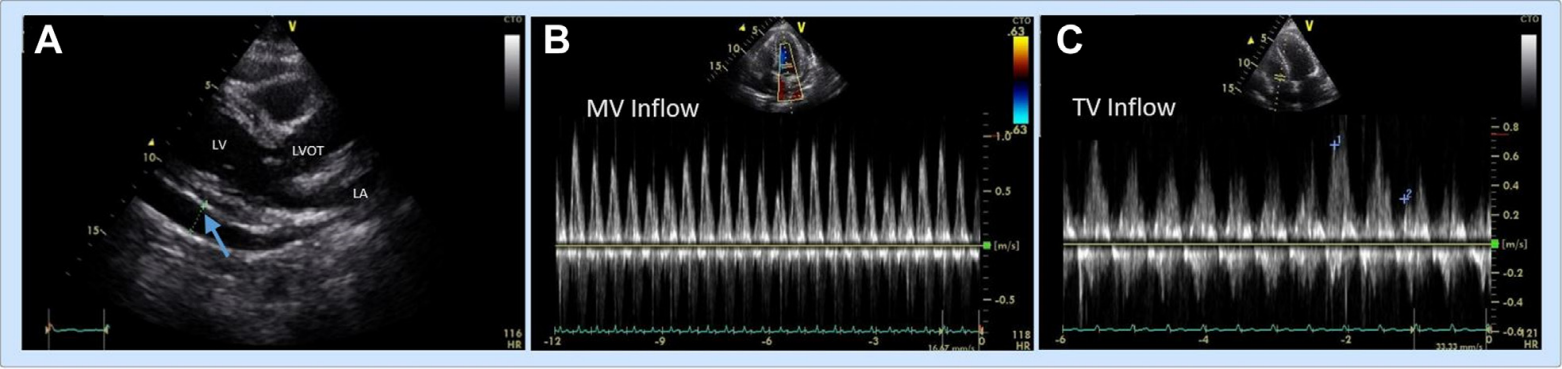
Echocardiographic Findings (A) Echocardiogram on initial presentation. A large pericardial effusion is seen on the long-axis view (blue arrow), left ventricle (LV), left atrium (LA) and left ventricle outflow tract (LVOT) shown. (B, C) Respiratory variation across the mitral valve (MV) and tricuspid valve (TV). The mitral valve peak percentage difference is 48.4%, and the tricuspid valve peak percentage difference is –119.4%.
